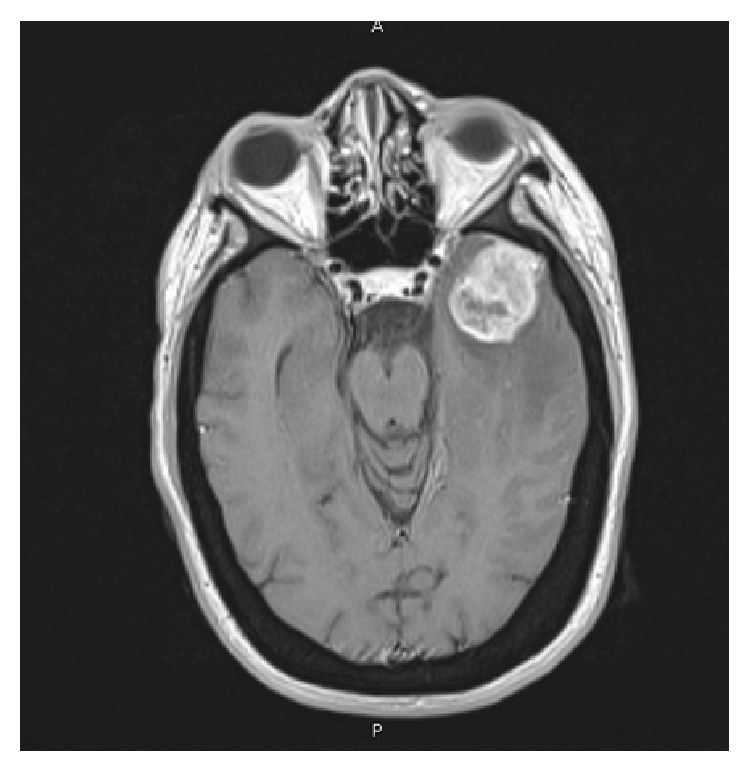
Axial T1-WI MRI demonstrating mass effect and infiltration to the temporal lobe with vasogenic edema.
